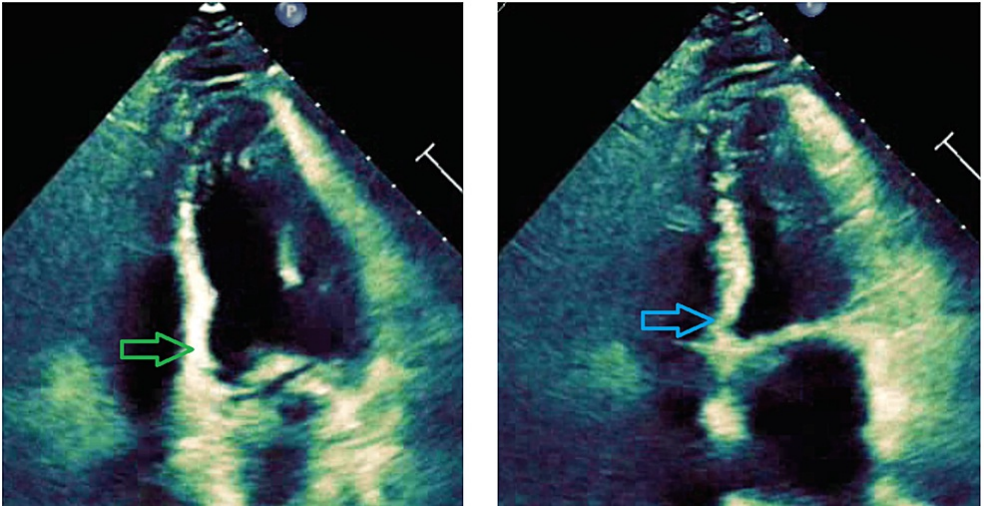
Transthoracic echocardiogram apical 4 -chamber view showing akinesia of the basal inferoseptal wall. Abnormal motion during diastole (green arrow) and systole (blue arrow)
